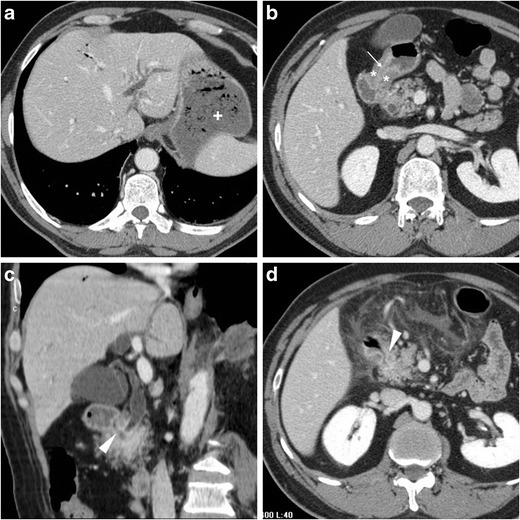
In a 53-year-old male with longstanding history of untreated duodenal ulcer, contrast-enhanced CT (a...c) revealed overdistended stomach (+) with fluid, normal-appearing gallbladder, minimal intrahepatic biliary dilatation in left liver lobe and some air in peripheral intrahepatic ducts (arrows) without previous surgery or biliary intervention. The antropyloric tract showed oedematous submucosa (*) and enhancing mucosa (thin arrow in b), and closely adhered to the ventral aspect of pancreatic head, and a thin fluid-containing track suggesting fistulisation (arrowhead in c) was identifiable between proximal duodenum and distal choledochus. Endoscopy showed duodenal bulb deformation from chronic PUD without active ulcers, and endoscopic retrograde cholangiopancreatography was unsuccessful. During a bout of acute pancreatitis, repeated CT (d) confirmed choledocho-duodenal fistula (arrowhead). Prolonged medical therapy ultimately allowed resolution of symptoms [Adapted with permission from ref. no [22]]
